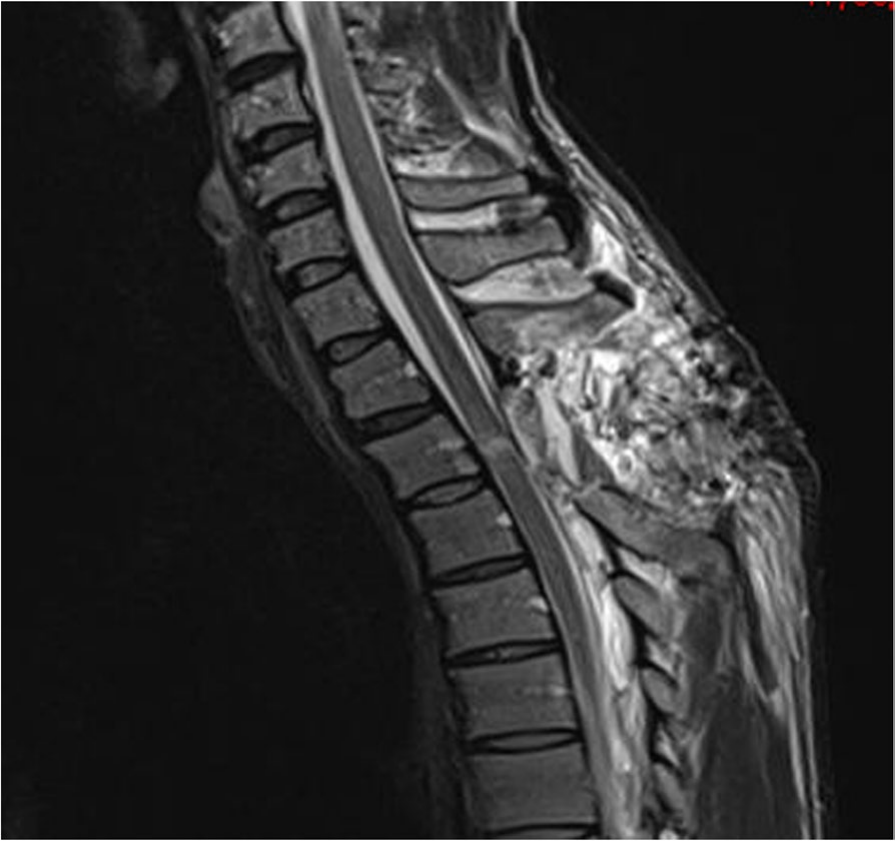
Immediate Post-operative MRI T2 weighted lateral view demonstrating the high signal in the repaired cord and soft tissue changes
